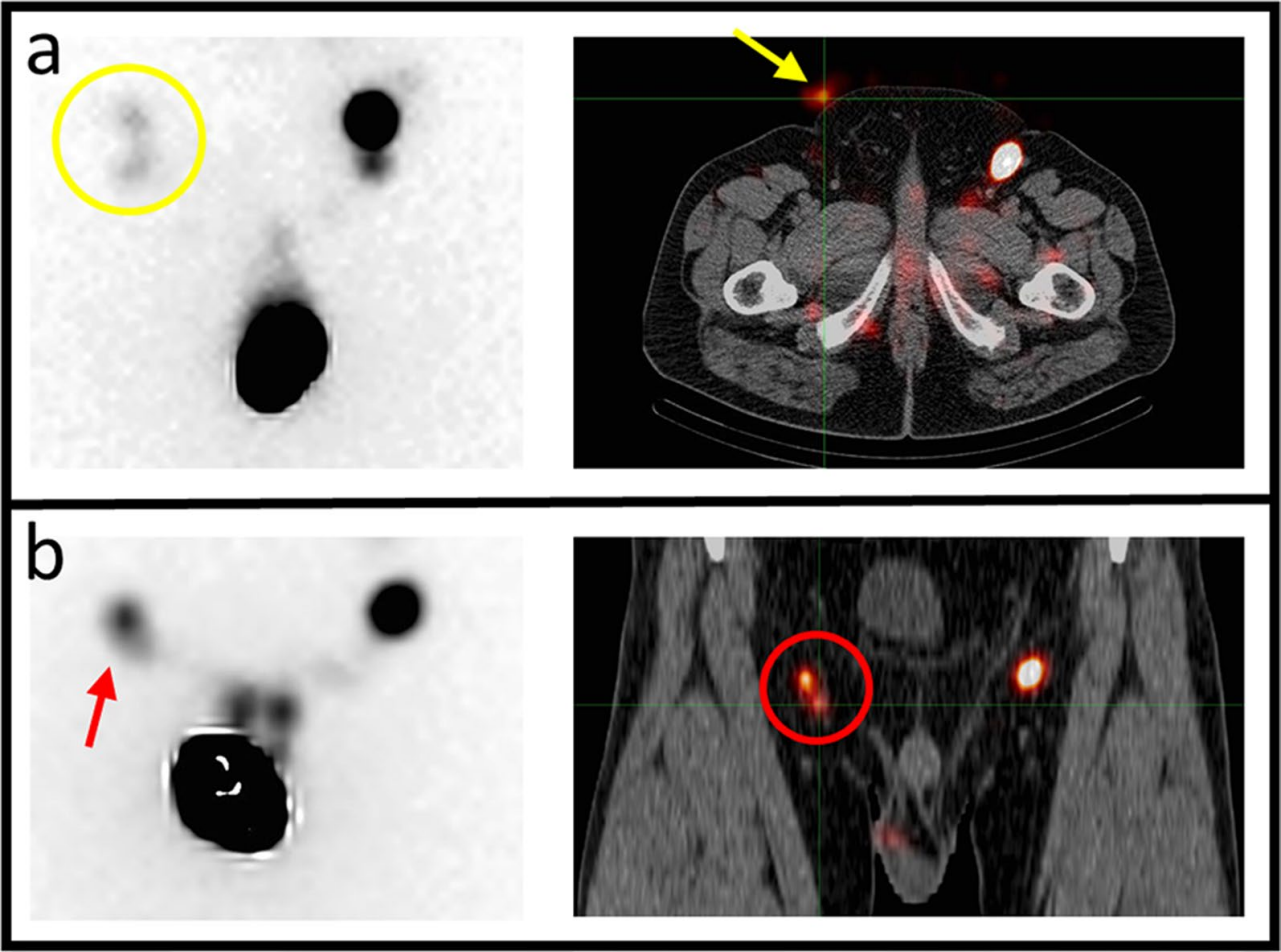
Discrepant findings between static planar and SPECT/CT imaging. a: static planar (yellow circle) > SPECT/CT image, falsely higher due to contamination on the skin (yellow arrow). b: static planar < SPECT/CT image (red circle), due to superposition (red arrow) of two SNs on the static planar image
